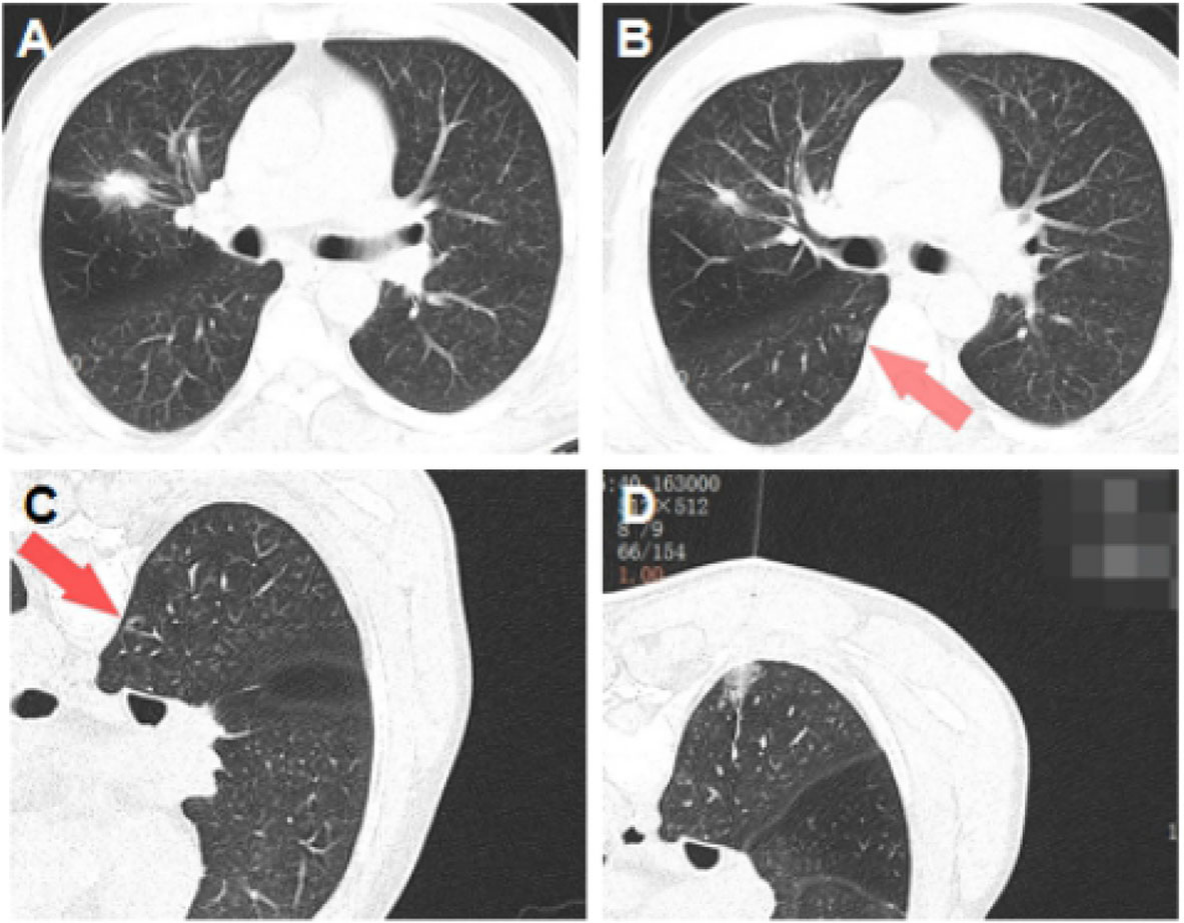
a The SPN located at the right upper lobe; b The red arrow indicates the GGO that was located at the right lower lobe; c The patient received localization procedure in prone position; d CT scan after localizer placement, from which we can perceive the tip of the hook wire was too close to a a side branch of basal segmental pulmonary artery. SPN, solid pulmonary nodule; GGO, ground-glass opacity; CT, computed tomography
